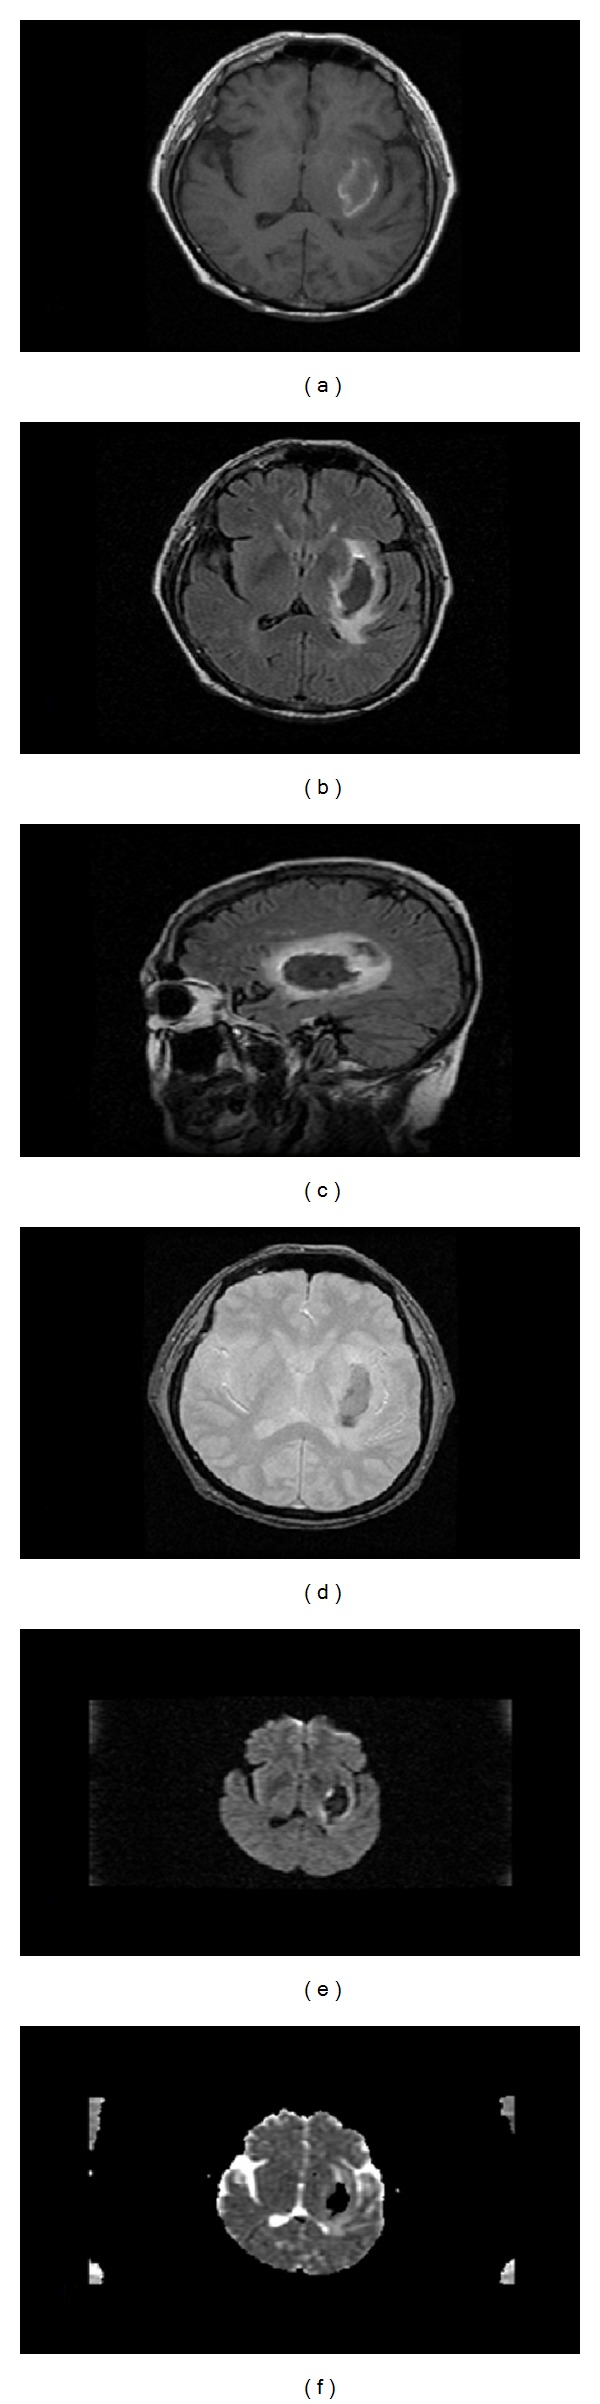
Brain magnetic resonance imaging showing a left intracranial hemorrhage. (a) Axial T1 weighted image, (b) axial T2 weighted image, (c) sagittal T1 weighted image, (d) axial gradient echo (GRE) image, (e) axial diffusion weighted image, (f) adjusted diffusion coefficient (ADC) image.
